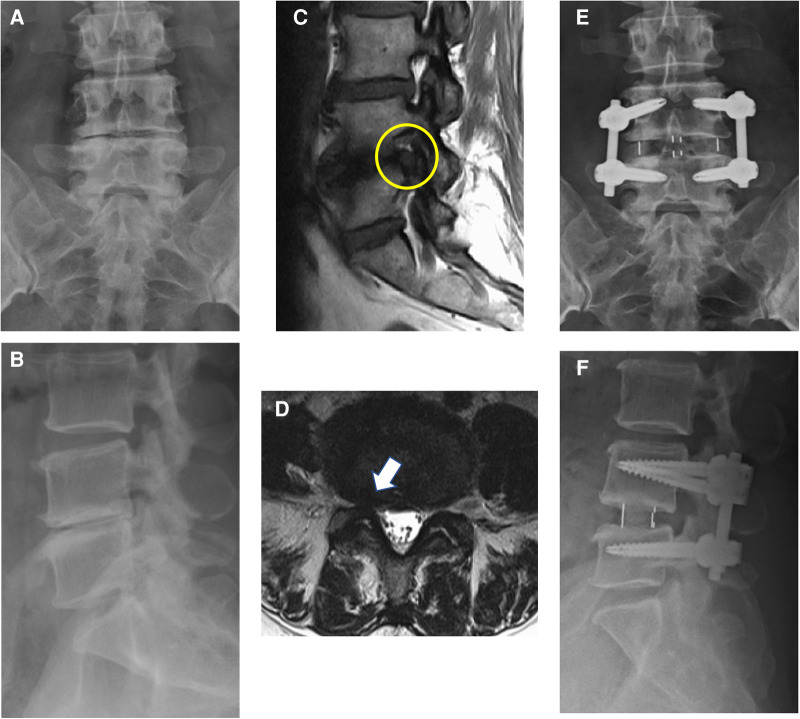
Anterior–posterior view (A) and lateral view (B) of a 67-year-old woman with degenerative disc disease. Sagittal T1-weighted MRI (C) and axial T2-weighted MRI (D) showed foraminal stenosis of L4/L5 (yellow circle and white arrow). Postoperative anterior–posterior view (E) and lateral view (F) of this patient. OLIF, L4/L5, with posterior instrumentation was done.
